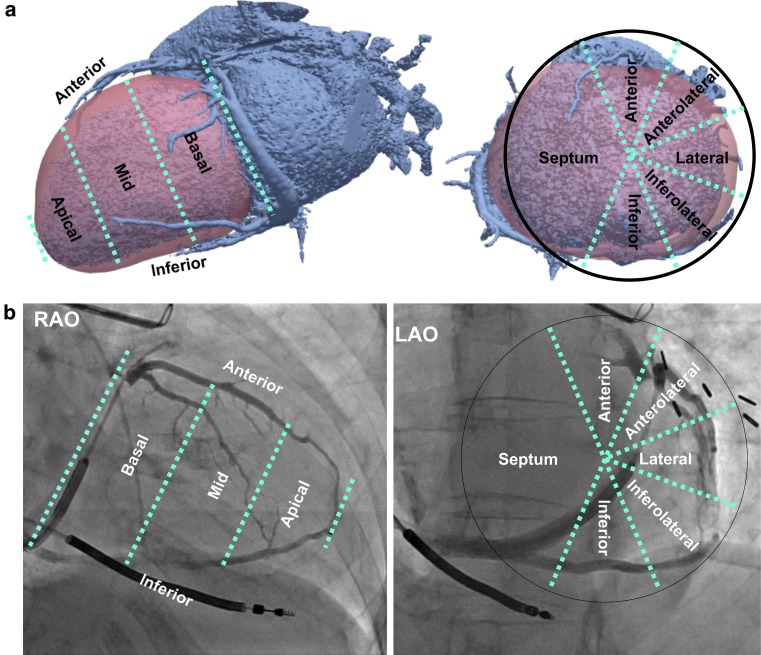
Coronary venous classification used in the present study shown on 3D computed tomography angiography (CTA) images (a) and 2D fluoroscopic angiography (FA) images of patient 14 (b). *LAO* left anterior oblique, *RAO* right anterior oblique
